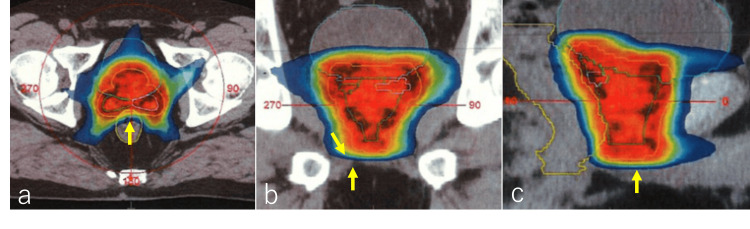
Intensity-modulated radiation therapy Intensity-modulated radiation therapy delivered a total dose of 78 Gy in 39 fractions. Axial (a), coronal (b), and sagittal (c) images are presented, highlighting the tumor-infiltrating lesion in the muscle on the right side of the prostatic apex, as previously identified in CT and MRI scans, which was targeted for irradiation with a submarginal approach (indicated by arrows in b).
